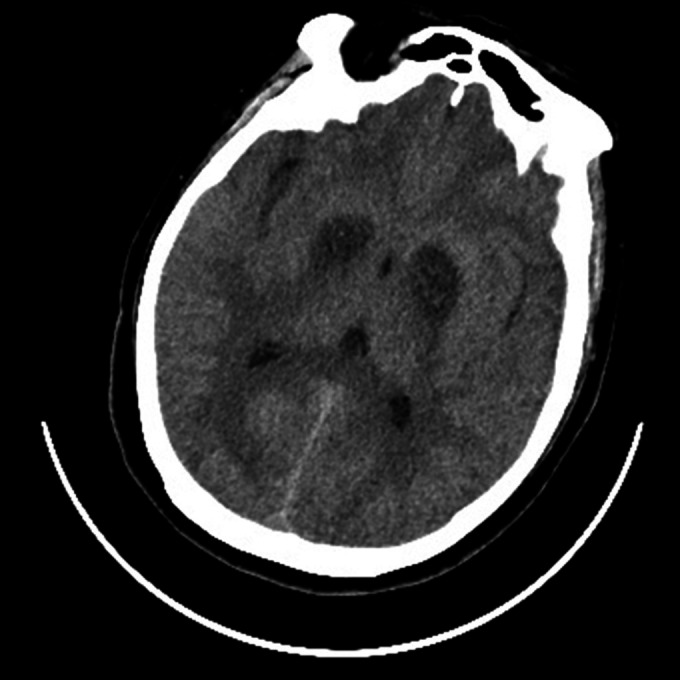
Axial computed tomography scan of the brain showed symmetrical hypoattenuation of the bilateral basal ganglia.
